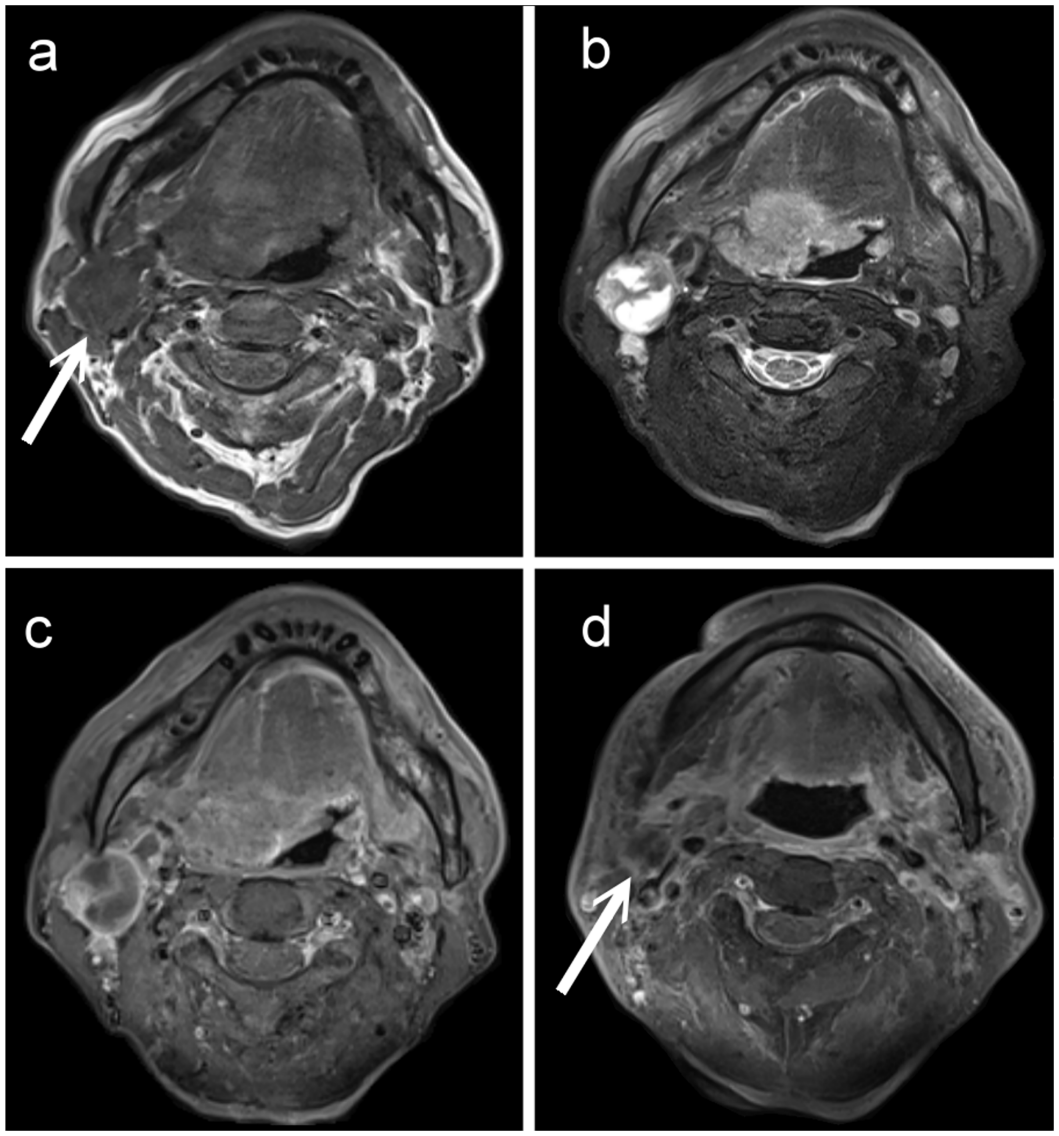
A 54-year-old male patient with right tongue base oropharyngeal squamous cell carcinoma and necrotic nodal metastasis. (A) T1-weighted, (B) fat-suppressed T2-weighted, and (C) contrast-enhanced fat-suppressed T1-weighted MRI images show an enlarged necrotic lymph node (arrow) in the right neck level IIA with irregular walls and central complex fluid-like content. (D) Contrast-enhanced fat-suppressed T1-weighted MRI three months after completion of chemoradiotherapy shows a residual necrotic node (arrows). Subsequent echo-guided fine needle aspiration cytology yielded metastatic carcinoma. The patient also experienced lung metastasis at a later date.
